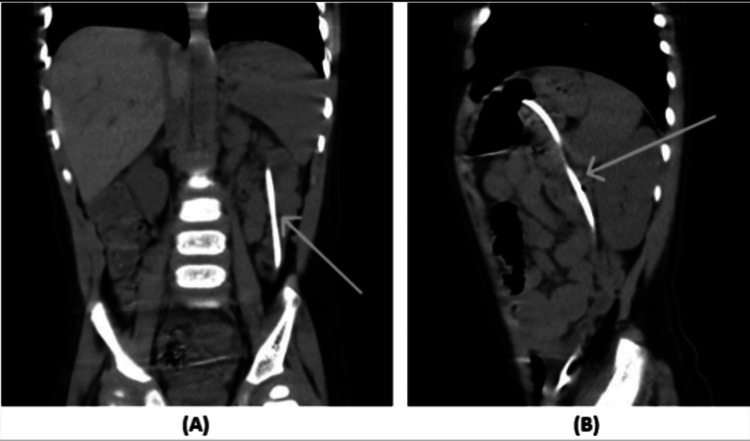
Plain abdominal CT scan (A) Coronal and (B) Sagittal sections showing the ventriculoperitoneal shunt invasion through the transverse colon.
